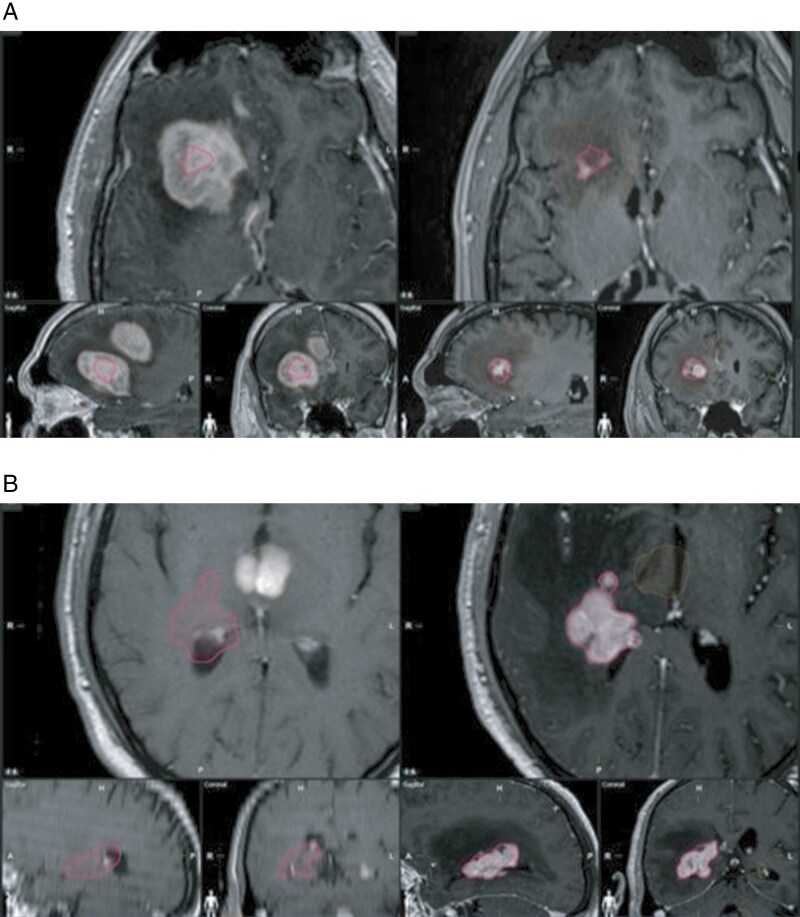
(A) Local versus distant progression of PCNSL. (A) is an example of local tumor progression on MRI T1-post contrast (left pre-HDMTX; right post-HDMTX). The black borders correlate with the lesion after progression. (B) Local versus distant progression of PCNSL. (B) is an example of distant tumor progression on MRI T1-post contrast (left pre-HDMTX; right post-HDMTX). The black borders correlate with the lesion after progression.
